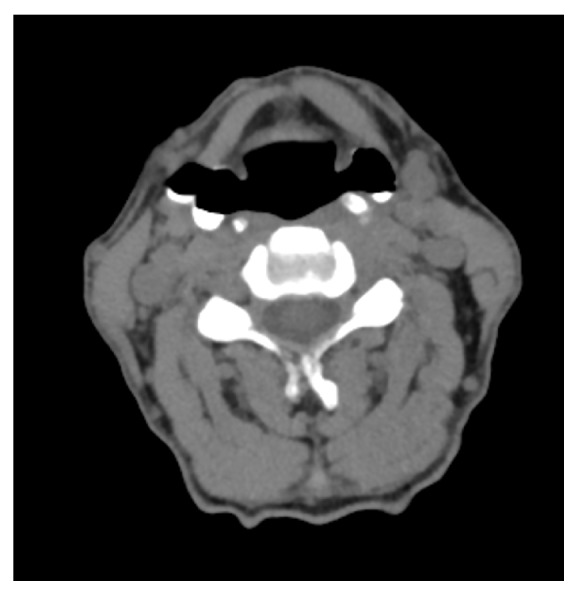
Noncontrast CT scan of the neck showing the bilateral pouches.
